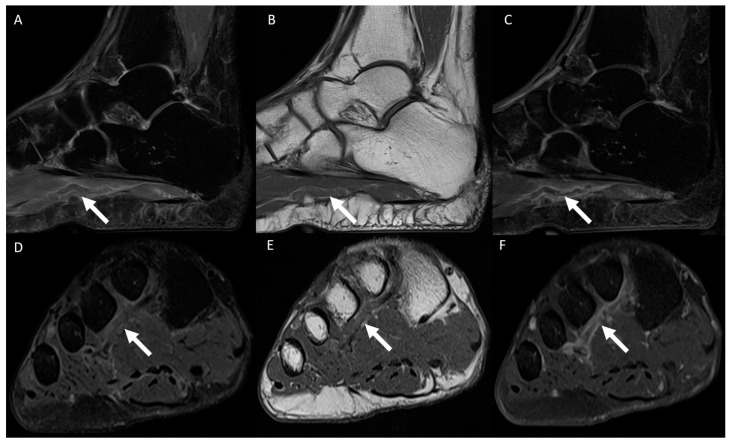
A 71-year-old woman presented with plantar pain of her right foot while walking for the past 7 days. Sagittal MRI images of the ankle, including a T2-weighted fat-suppressed scan (A), a T1-weighted scan (B), and a post-gadolinium T1-weighted fat-suppressed scan (C), revealed thrombosis in the lateral plantar vein, identified by venous dilation, with intraluminal thrombus (arrow in (A,B)) and an intravenous filling defect (arrow in (C)). Additionally, the short-axis plane MRI images of the forefoot, including a T2-weighted fat-suppressed scan (D), a T1-weighted scan (E), and a post-gadolinium T1-weighted fat-suppressed scan (F), exhibited thrombosis in the plantar venous arch, characterized by venous enlargement with intraluminal thrombus (arrow in (D,E)) and an intravenous filling defect (arrow in (F)).
